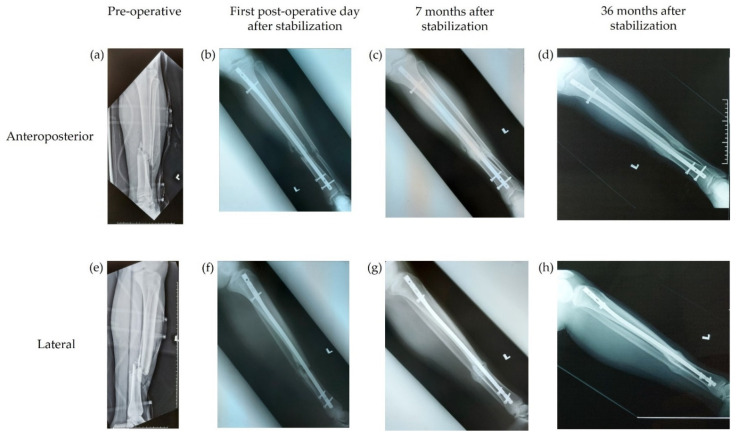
Example of timely bony union, as assessed by the independent experts. Radiographs from a 64-year-old male patient with a Gustilo type IIIa open tibial fracture. (a–d) Anteroposterior radiographs. (e–h) Lateral radiographs. (a,e) Radiographs taken pre-operatively; (b,f) radiographs taken on the first post-operative day after stabilization of the fracture; (c,g) radiographs taken 7 months after stabilization and demonstrate bony union; and (d,h) radiographs taken 36 months after stabilization.
